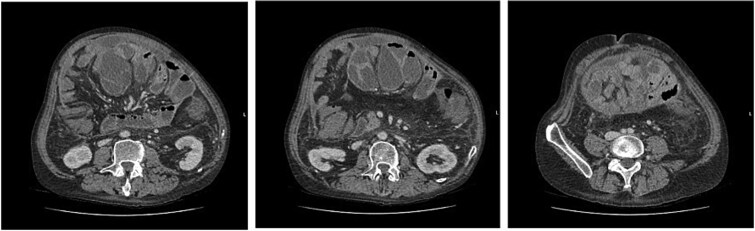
(left to right–cranial to caudal) Axial contrast-enhanced CT images demonstrating dilated small bowel loops in the central abdomen, encapsulated in thickened peritoneum and with trace free fluid. Appearances are consistent with small bowel obstruction.
